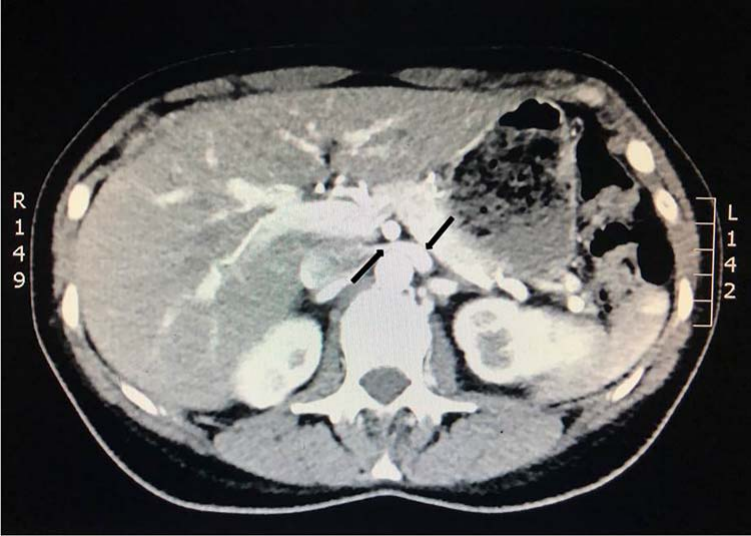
Computed tomography angiogram 6 months later showing pre-compressed LRV (right arrow); and compressed LRV (left arrow), but resolution of the congestion and swelling of the left kidney.
